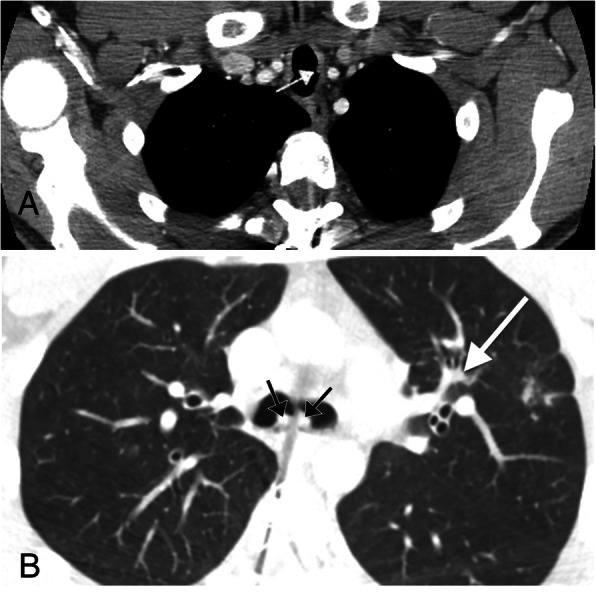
Recurrent respiratory papillomatosis. a Axial chest CT showing a soft tissue attenuation polyp in the lateral wall of the upper thoracic trachea (arrow). b Axial CT image of the same patient at the level of the carina showing two additional soft tissue attenuation polyps (black arrows). This image also demonstrates bronchiectasis in the left upper lobe which occurred secondary to luminal obstruction of a distal airway secondary to a polyp (white arrow)
